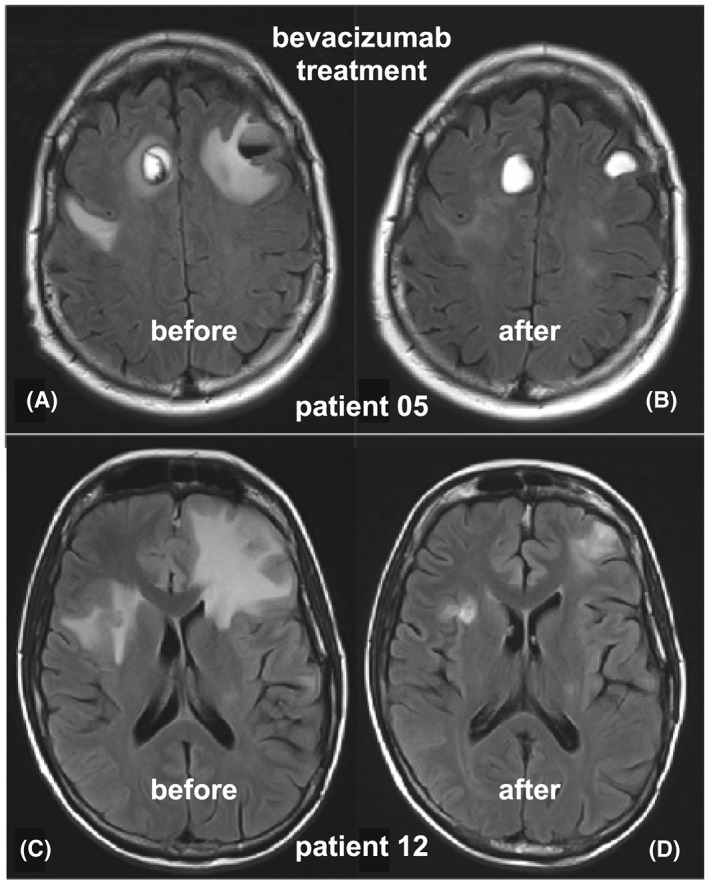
Radiological improvement in peritumoral edema after bevacizumab. Axial FLAIR images for P05 before (A) and after (B) bevacizumab show a marked decrease in the edema surrounding hemorrhagic bilateral frontal lobe metastases. The milder FLAIR hyperintensity further posteriorly was attributed to prior treatment (including WBRT). Axial FLAIR images for P12 before (C) and after (D) bevacizumab demonstrated marked improvement in edema without any use of corticosteroids
